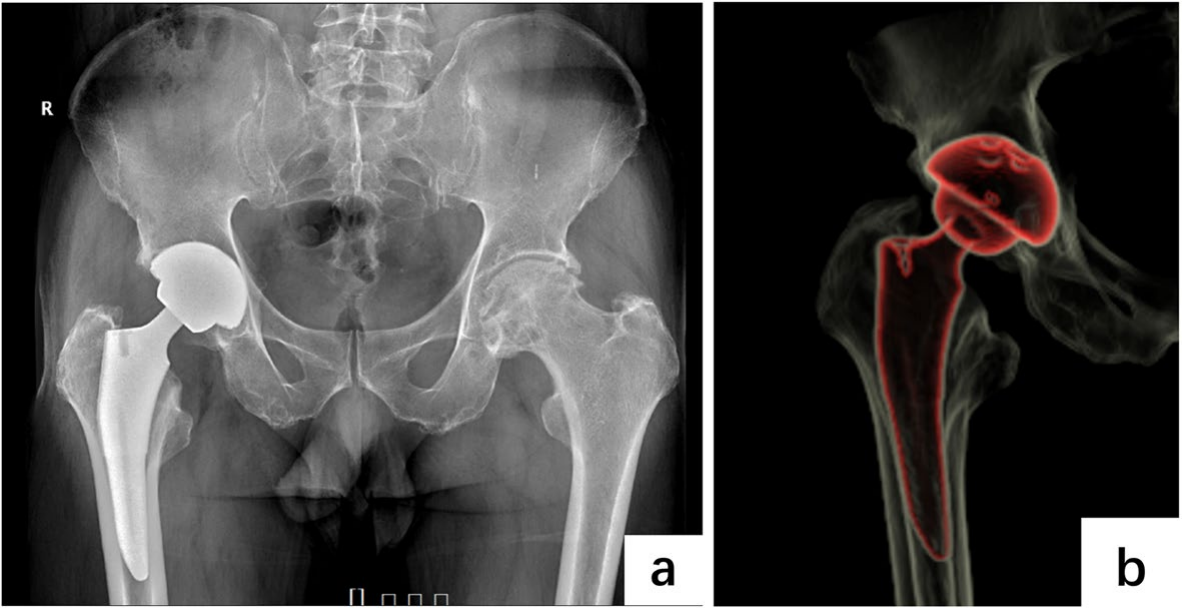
Presentation of spontaneous reduction of the dissociated liner eight months postoperatively.Plain pelvic radiograph shows no protrusion of the ceramic liner. a CT contour reconstruction (b) confirms proper seating of the liner in the acetabular cup (b)
